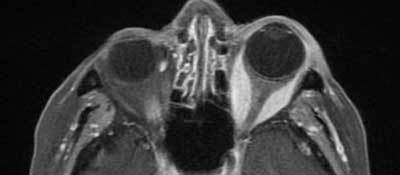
Contrast-enhanced fat suppressed axial T1-weighted MR-images of the orbit confirm fusiform thickening of the left medial and lateral muscles. No extension of intra-orbital changes into the cavernous sinus is seen.
